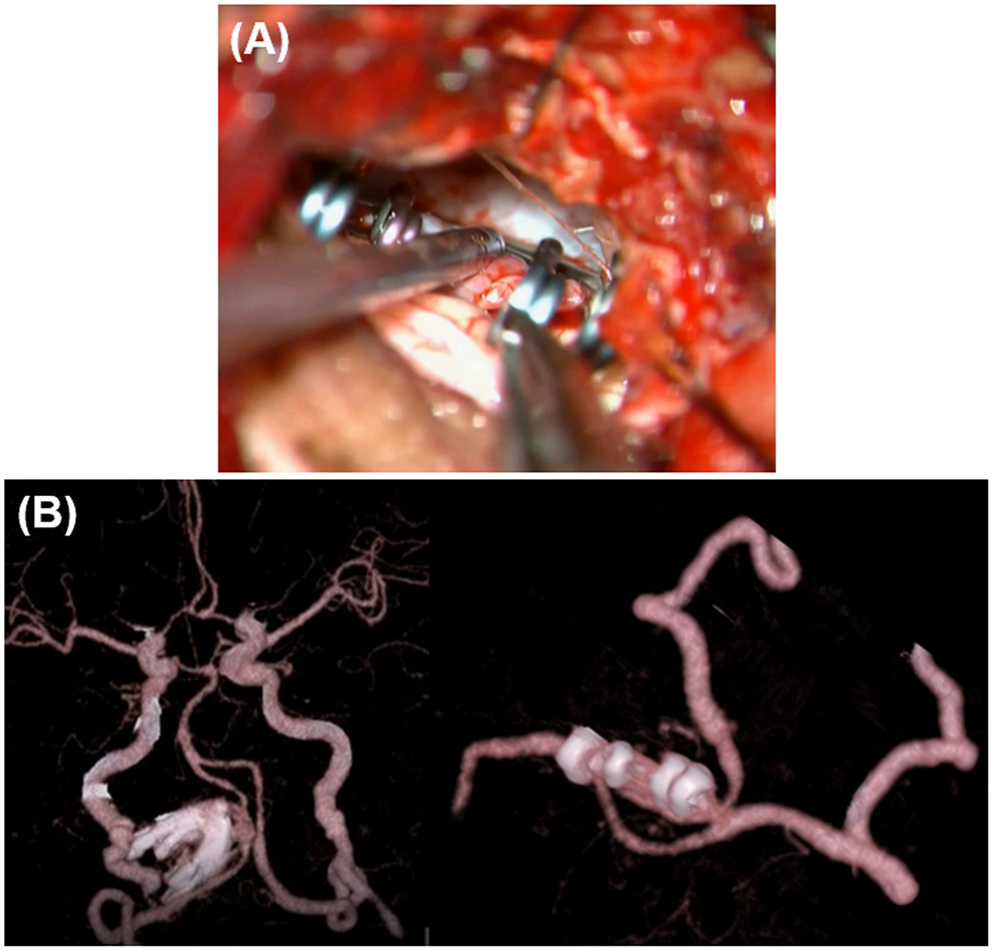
Intraoperative findings and postoperative computed tomography angiography (CTA) of case 1. (A) Intraoperative findings. (B) Postoperative CTA.
